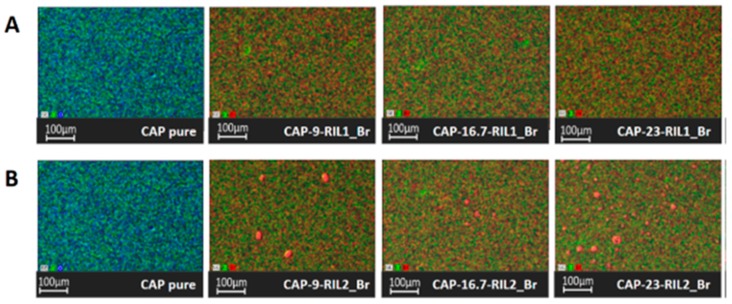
SEM- energy dispersive X-ray (EDX) images of film surface: (A) CAP and CAP-RIL1_Br and (B) CAP and CAP-RIL2_Br films (green—carbon atoms, red—bromine atoms, and blue—oxygen atoms).
